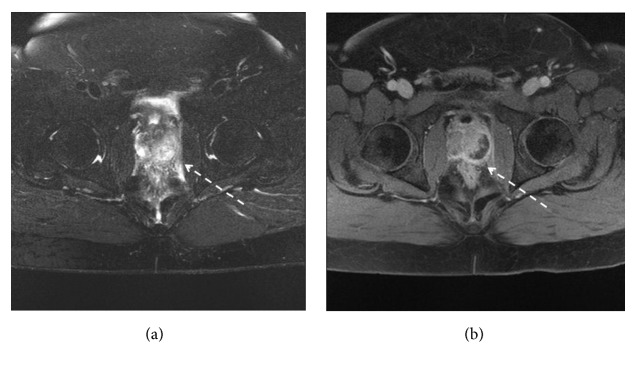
MRI of pelvis: axial FS T2WI (a), axial postcontrast T1WI (b). Before aspiration and antibiotic therapy showing fluid intensity lesion within posterolateral aspect of the gland measuring 24 × 12 × 14 mm with marginal enhancement (prostatic abscess).
